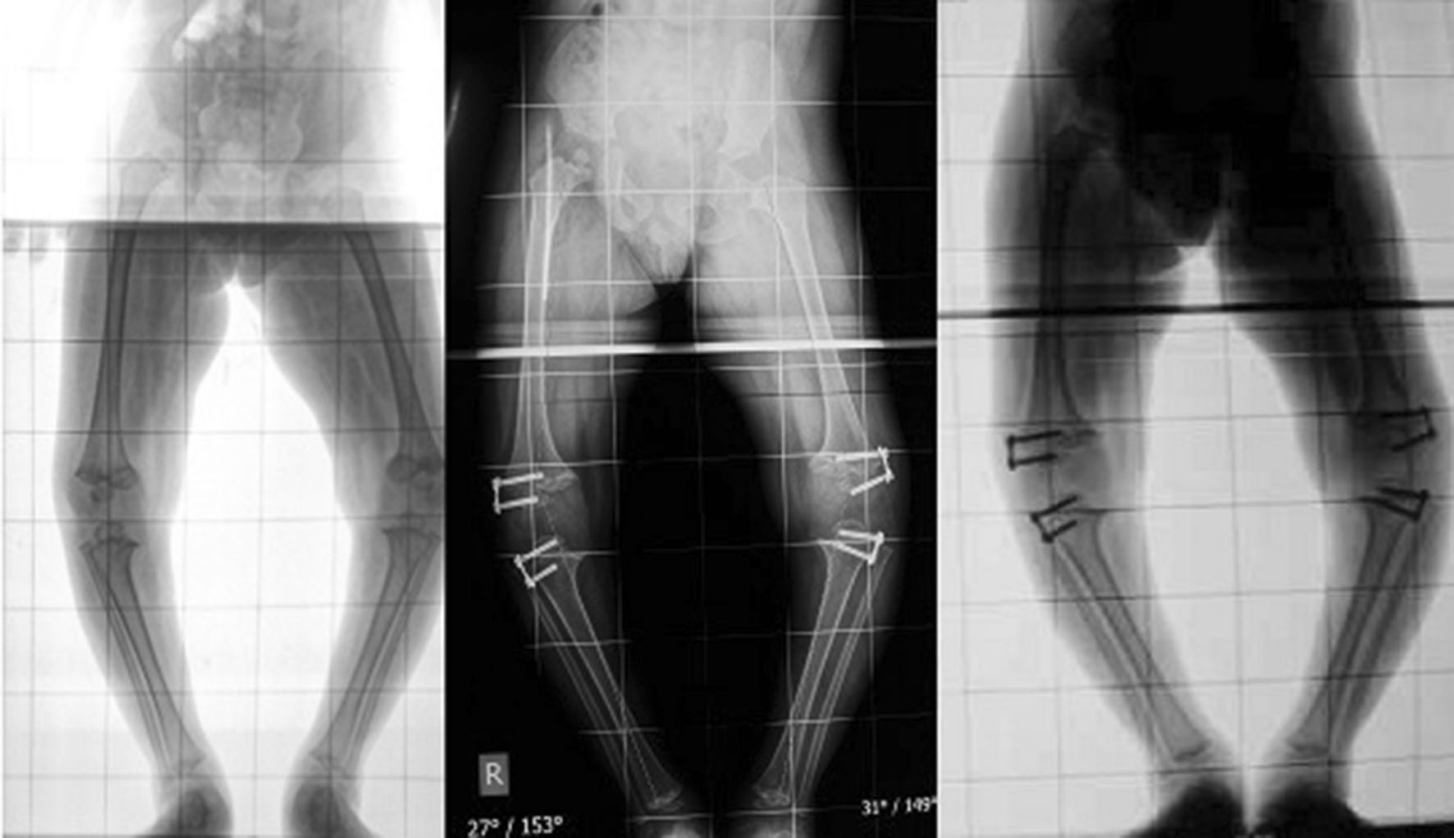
Radiographs showing failure of correction of deformity in a case of skeletal dysplasia managed with eight plate (8 months and 2 years post-operatively)
